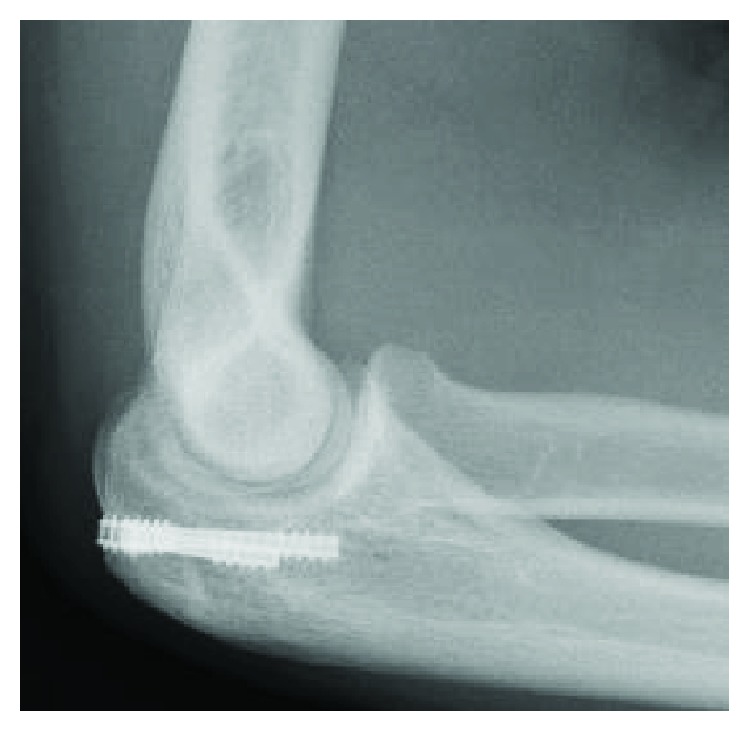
The radiographs of the right elbow one year after surgery.
